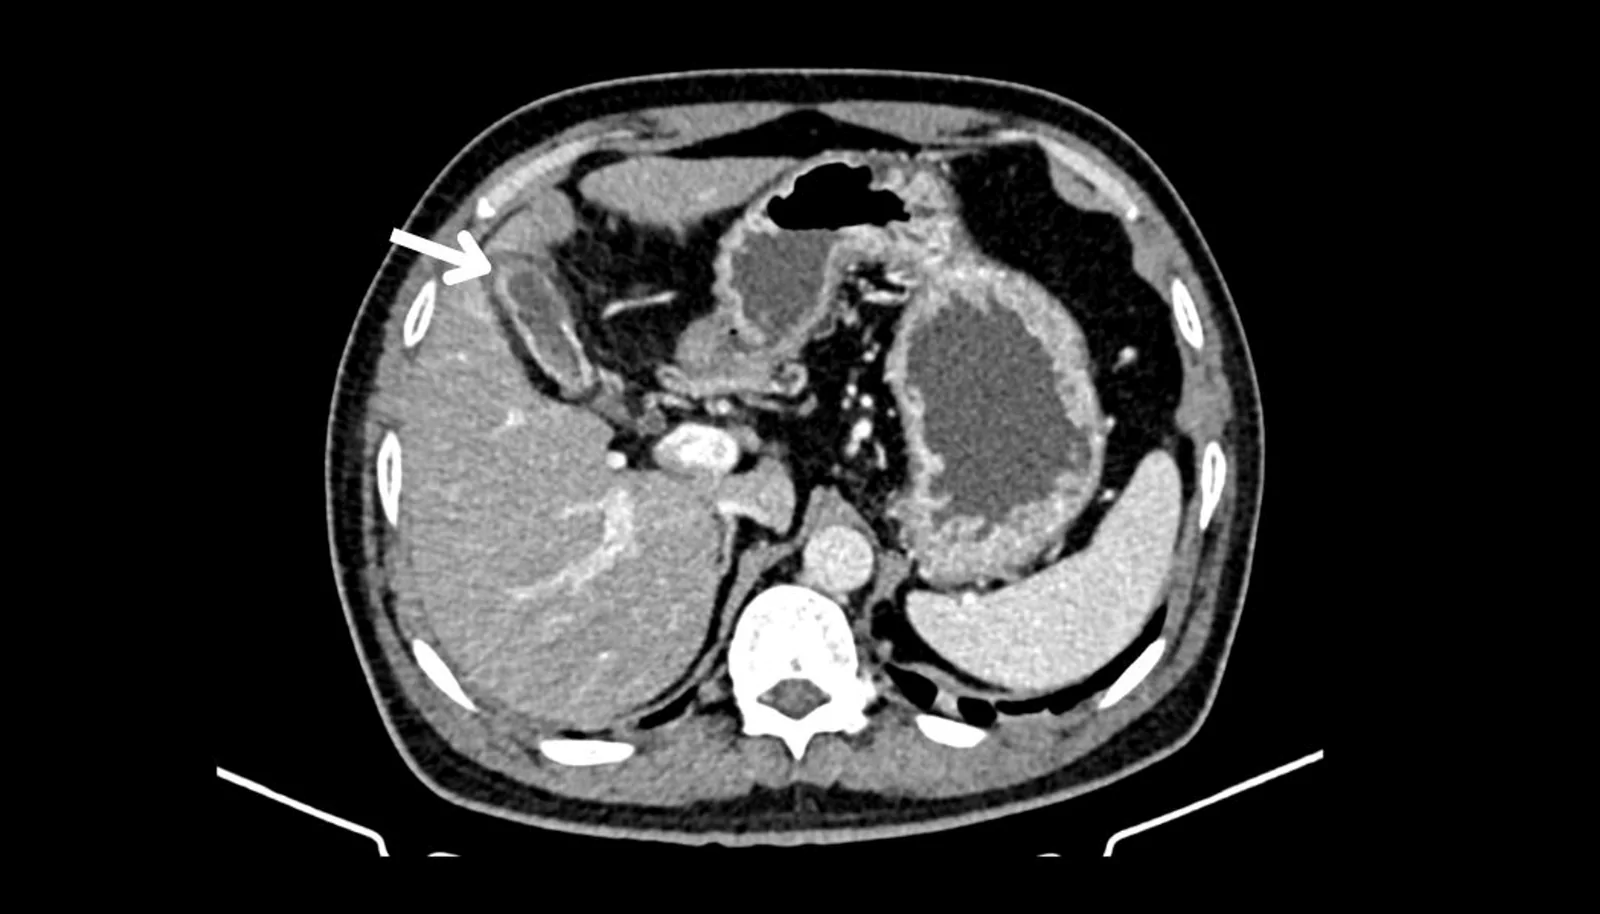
Axial section of the contrast-enhanced computed tomography (CECT) abdomen showing a thick-walled gallbladder with cholelithiasis The arrowhead points to the thickened gallbladder wall.
